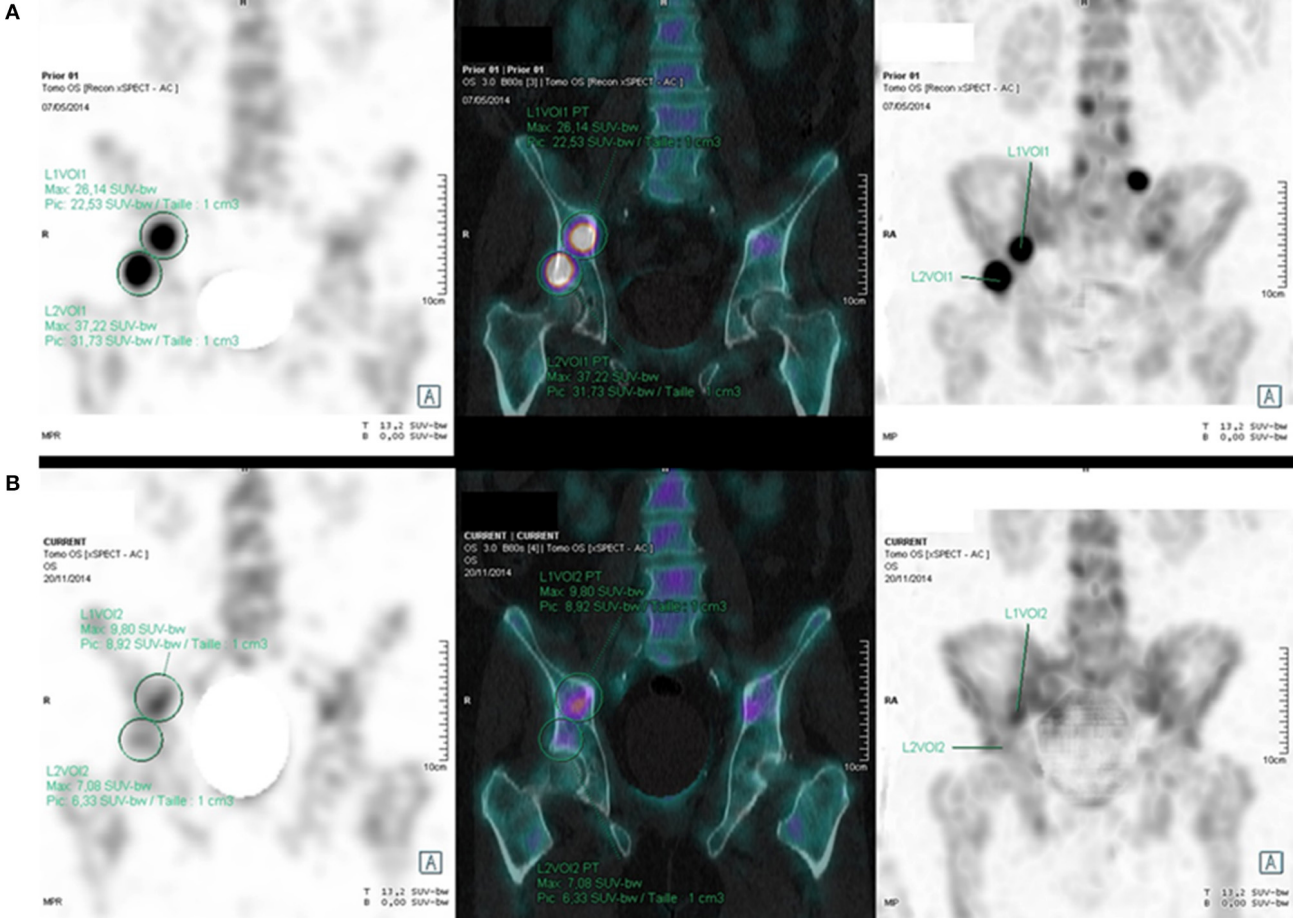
61 years old patient with BmCRPC; SPECT, SPECT-CT, and MIP images show a quantitative regression of the right acetabulum and iliac wing uptakes (SUVmax: respectively, 7.08 vs. 37.22, and 9.80 vs. 26.14) between M0 (A) and M6 (B) (delay: 6.5 months), with a 97.2% PSA regression (0.72 vs. 26 ng/mL).
